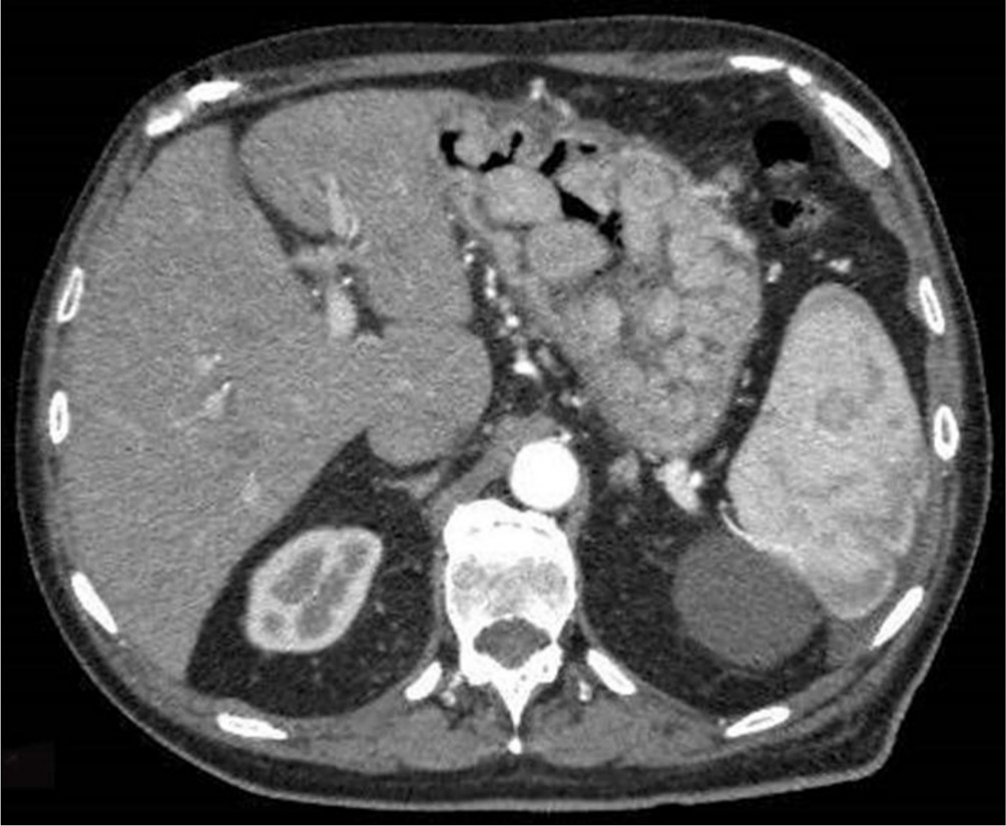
Abdominal CECT. Multiple solid masses in the stomach with early intense enhancement followed by progressive washout. CECT, contrast enhanced CT
